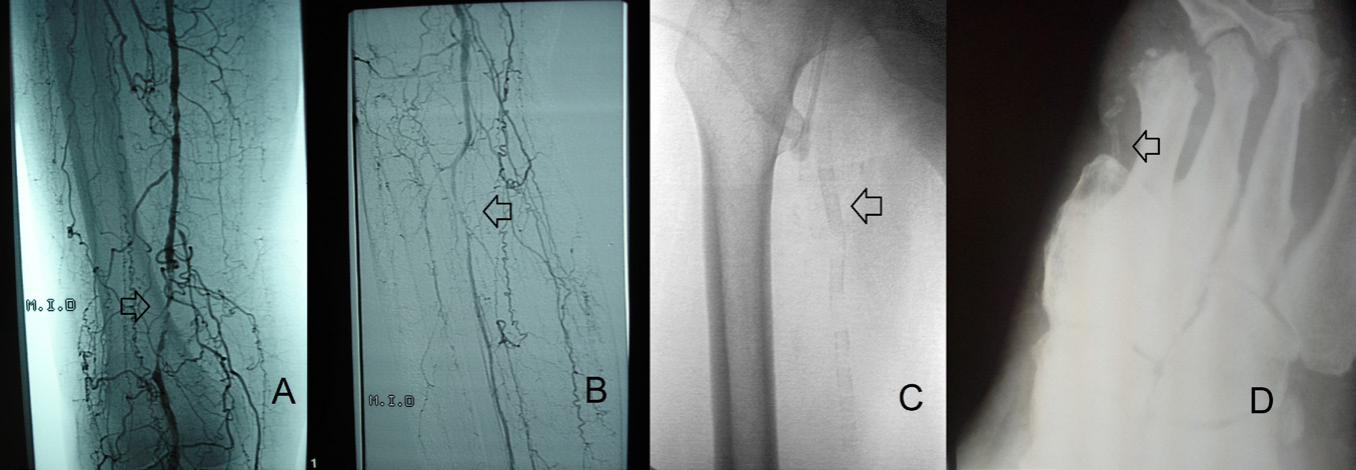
A & B) Digital arteriography with contrast showing images of arterial obstructions compatible with atherosclerosis (arrows). C & D) Radioscopy and X-ray without contrast showing images compatible with Monckeberg medial calcific sclerosis (arrows).
